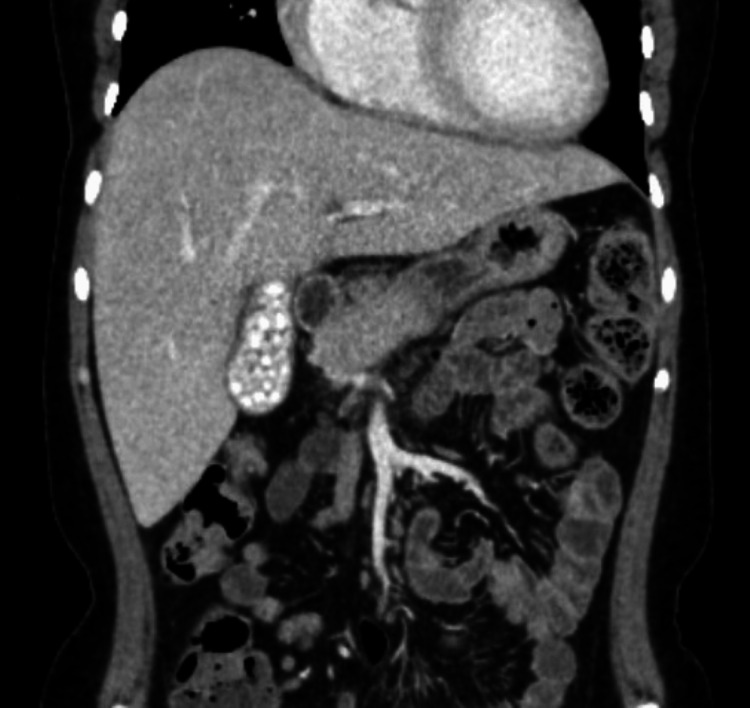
Coronal view of computed tomography of abdomen showing innumerable tiny calcified gallstones filling the entire gallbladder lumen (case 4).
